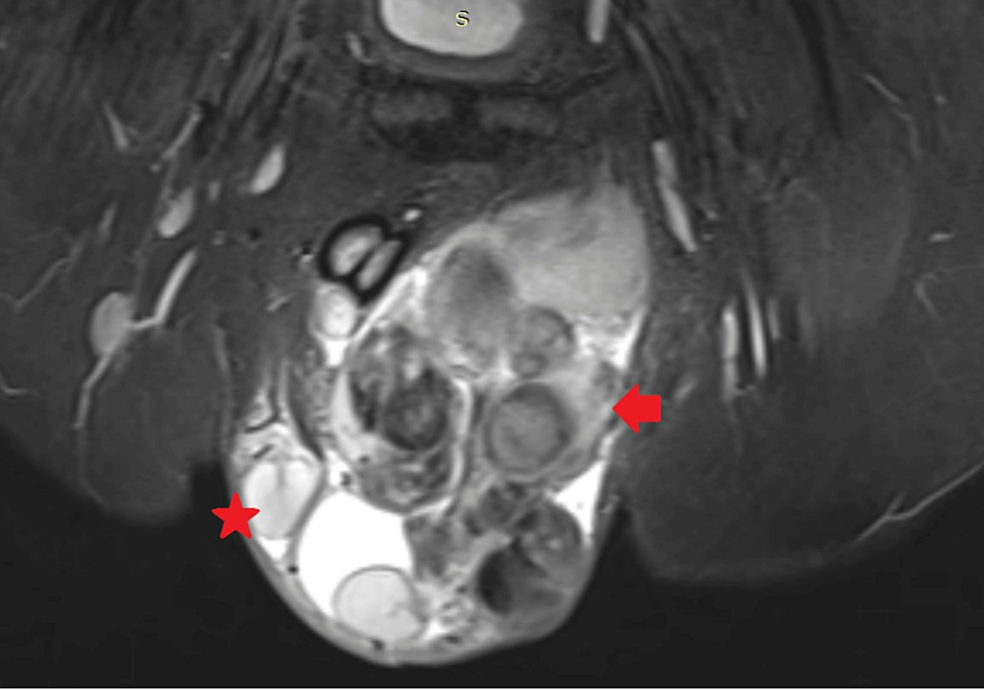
MRI T2 sequence in coronal section showing "*" (asterisk symbol) as the unaffected testis on the right side of the patient, and "→" (arrow head symbol) as the affected testis on the left side of the patient.
